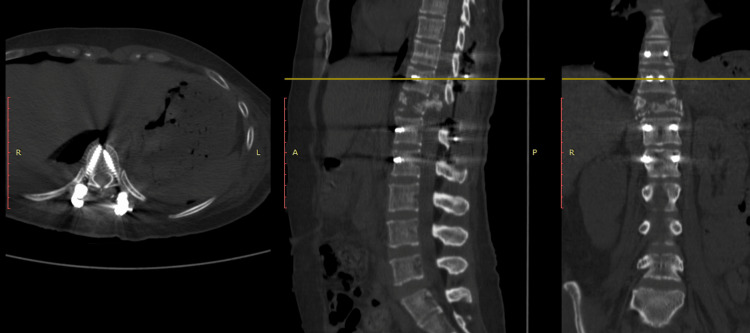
Case 5 postoperative control CT scan with axial, coronal, and sagittal views showing the transpedicular instrumentation from T10-T11 to L1-L2.
